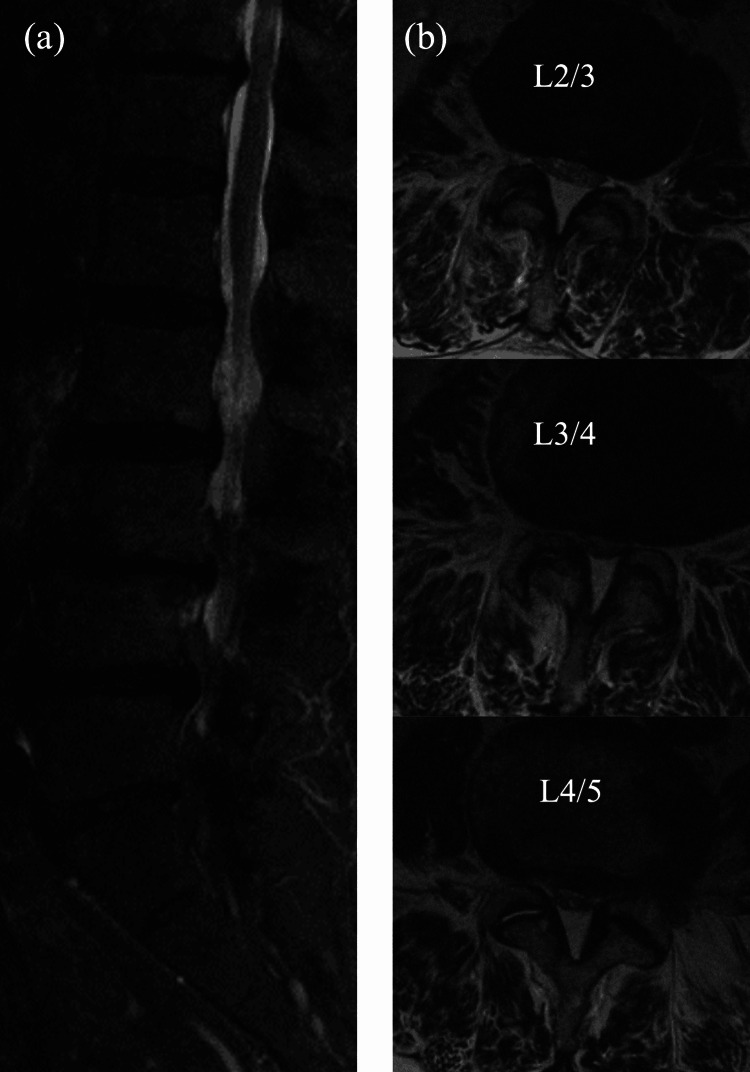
(a) Sagittal plane and (b) axial plane preoperative spinal MRI findings at L2/3, L3/4, and L4/5
